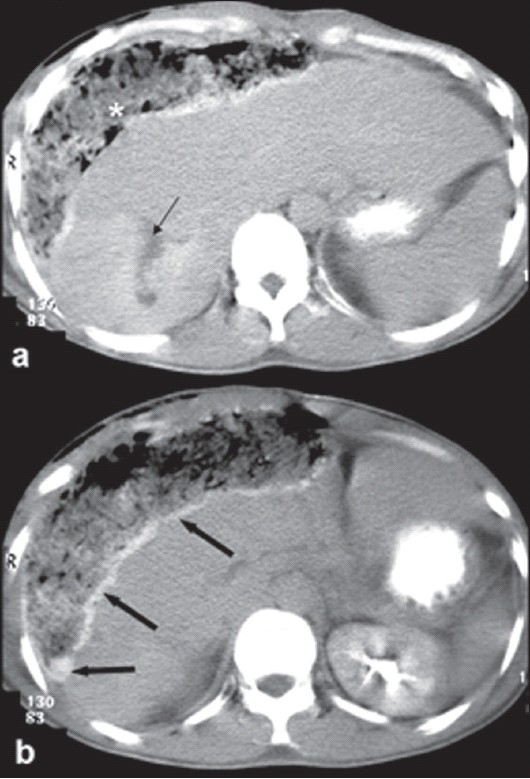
Delayed CT image (a) shows progressive centripetal filling-in of the tumor with contrast, confirming the diagnosis of a hemangioma, with a central non-enhancing area (arrow) suggestive of a fibrotic scar. Pack of sponges from the previous surgery (asterisk) can be seen at the liver surface. In addition, hyperdensity seen along the liver surface (solid arrows-b) represents active contrast extravasation
